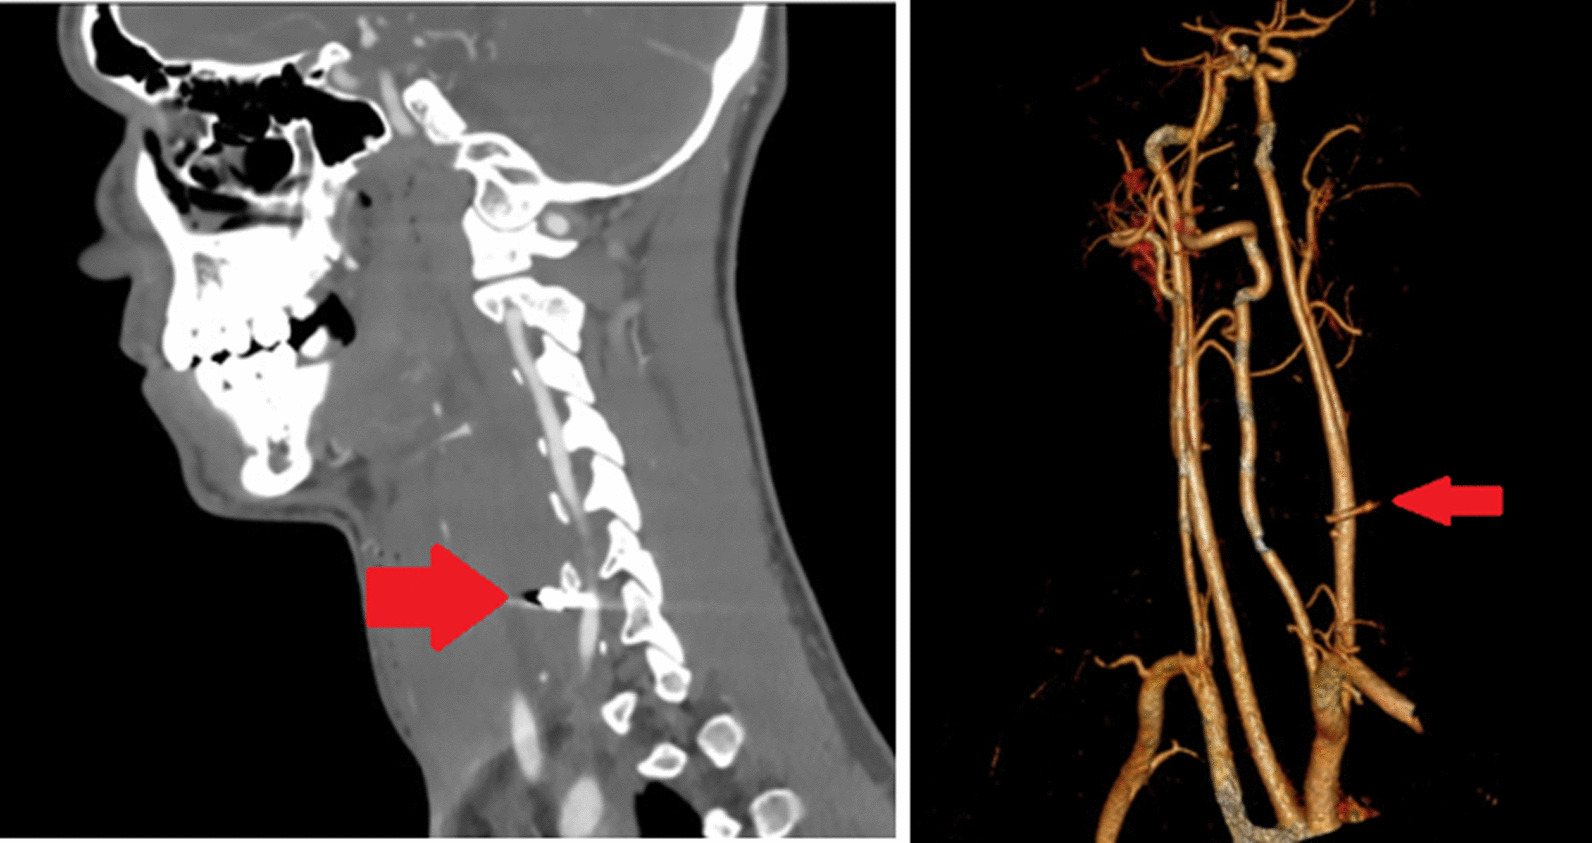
CT images and 3D reconstruction showing the projectile going through the anterior medial wall and exiting through the posterior wall of the carotid artery. Arrow demonstrating the pellet
